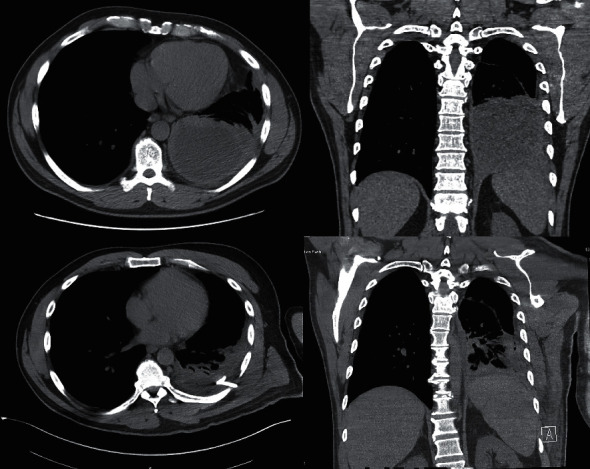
Chest CT showing left-sided pleural effusion, with follow-up chest CT demonstrating decreased fluid collection after systemic antibiotics and intrapleural fibrinolytic therapy.
